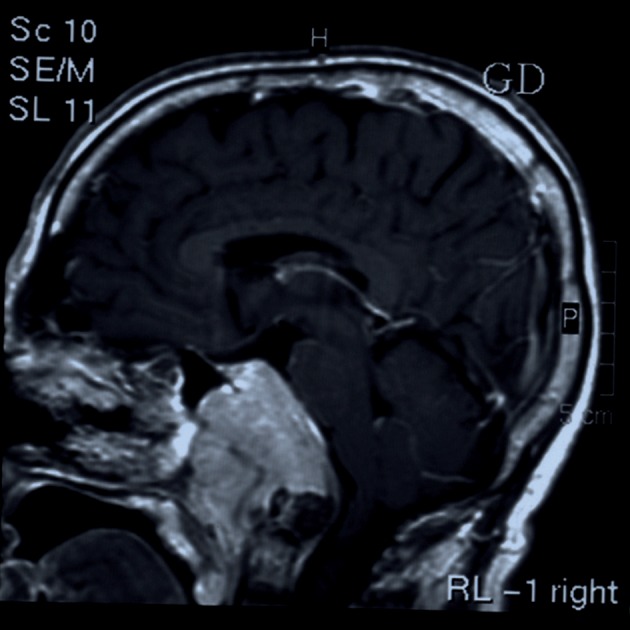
Sagital view in MRI; Nasopharyngeal mass with enhancement after gadolinium injection.
